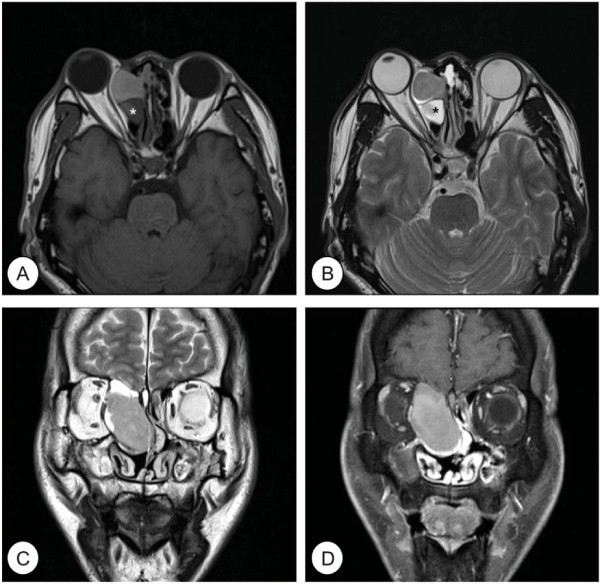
Preoperative magnetic resonance (MR) images of the paranasal sinus. MR images show a huge cystic mass displaying intermediate signal intensity on T1 axial (A), T2 axial (B), and T2 coronal images (C). Post-contrast T1 mage (D) show no enhancement of the cystic mass. Right ethmoidal sinusitis is noted (asterisk).
